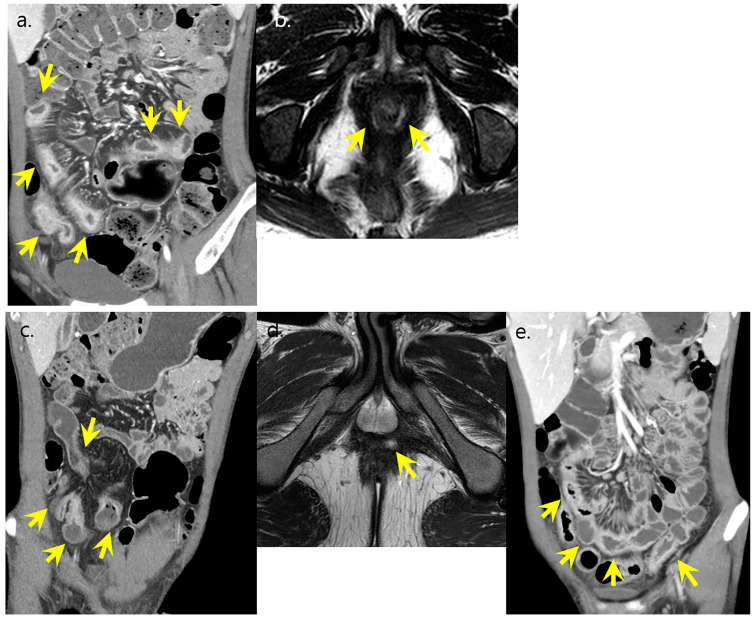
A 22-year-old male with Crohn’s disease showing unfavorable disease course. (a,b) Initial CT and MR showed severe active inflammation in entire ileum and ileocolon with active perianal lesion. (arrows) CT scores were graded as severe activity. (c,d) Follow-up exams after 2 years showed persisting active bowel inflammation and perianal lesion. (arrows) (e) CT activity was graded as severe due to persistent active bowel inflammation at the last follow up (arrows).
